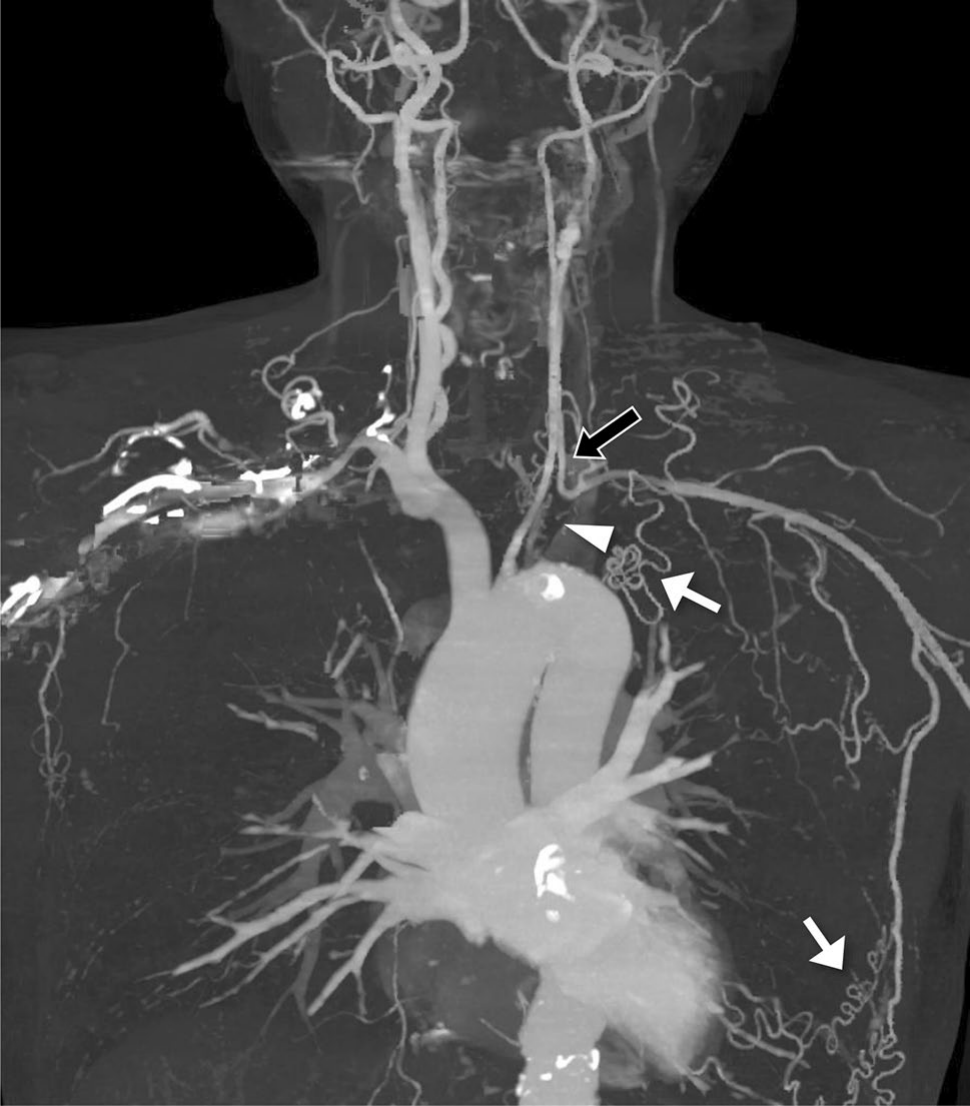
Subclavian steal in chronic-phase Takayasu arteritis in a 41-year-old woman. MIP of CT-angiography shows occlusion of the proximal portion of the left subclavian artery (arrowhead) with distal filling via the left vertebral (black arrow) and the intercostal arteries (white arrows)
